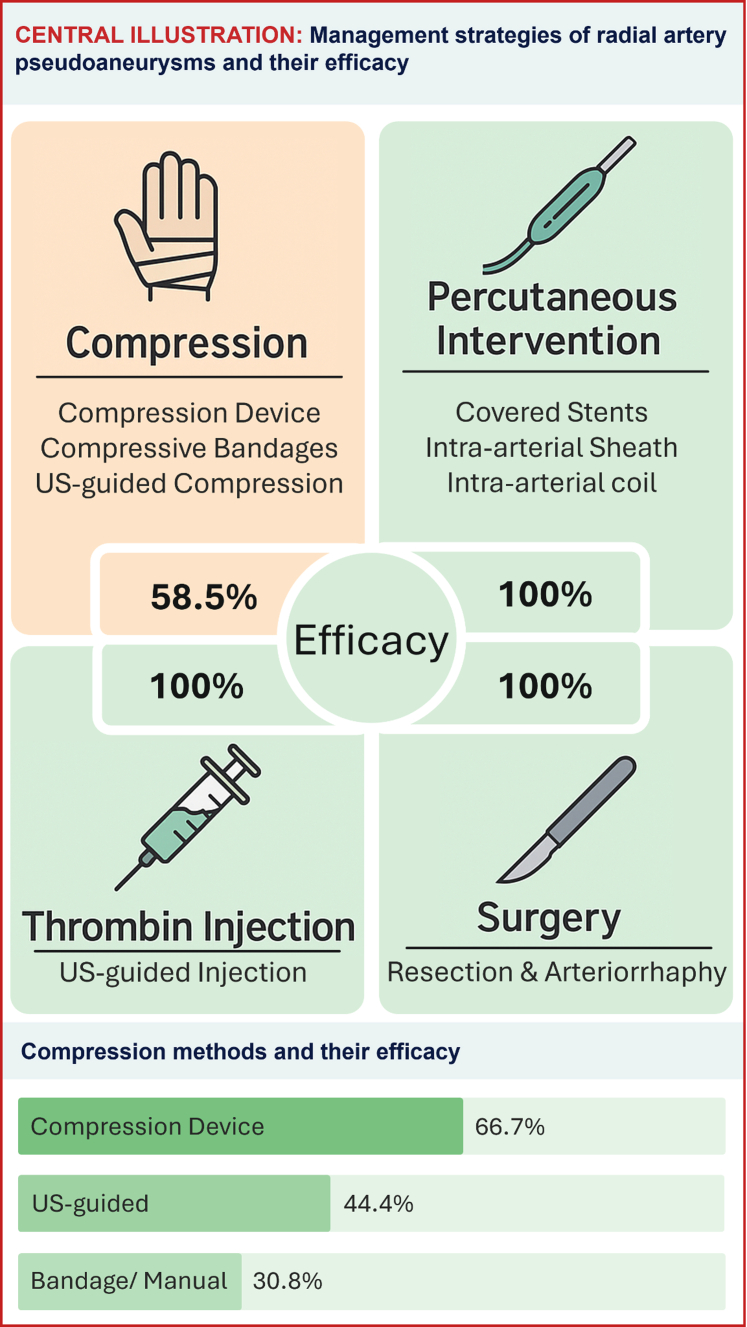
Management strategies of radial artery pseudoaneurysms (including the specific compression methods) and their efficacy. US, ultrasound.
